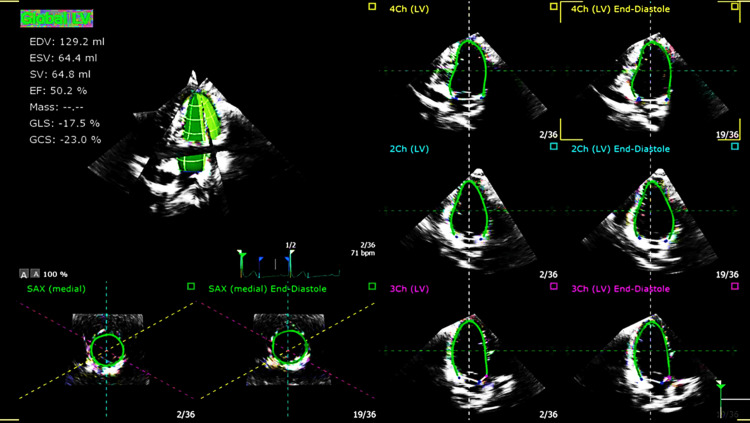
Transthoracic echo echocardiography showed the 3D full volume LVEF measurement which revealed an EF of 50%. 3D; 3 dimensional, LVEF; left ventricular ejection fraction, EF: ejection fraction.
